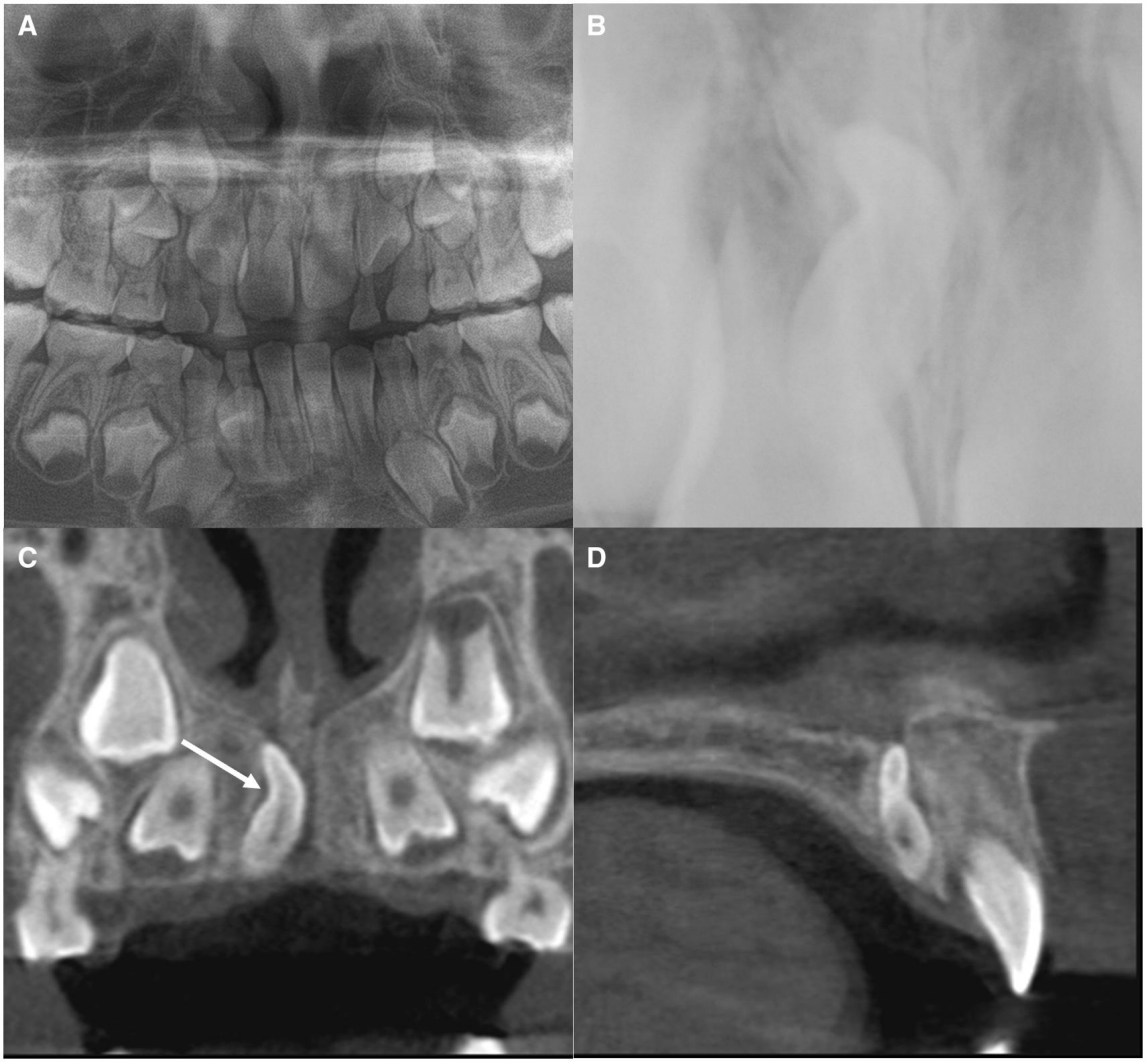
Dilaceration in the crown region in case 27. (A, B) Cropped panoramic and occlusal radiographs, respectively. (C, D) Cropped CBCT images (coronal and sagittal views, respectively). The arrow indicates the dilaceration in the crown region.
